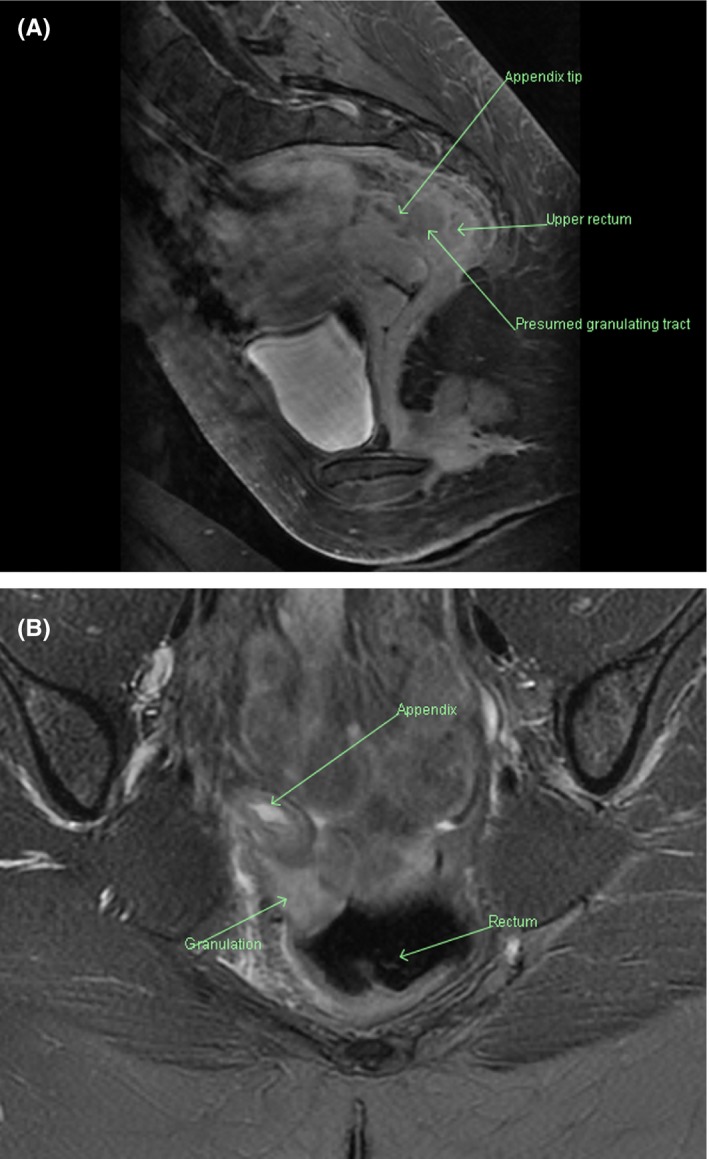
MRI Pelvis: (A) Sagittal View; (B) Axial View
